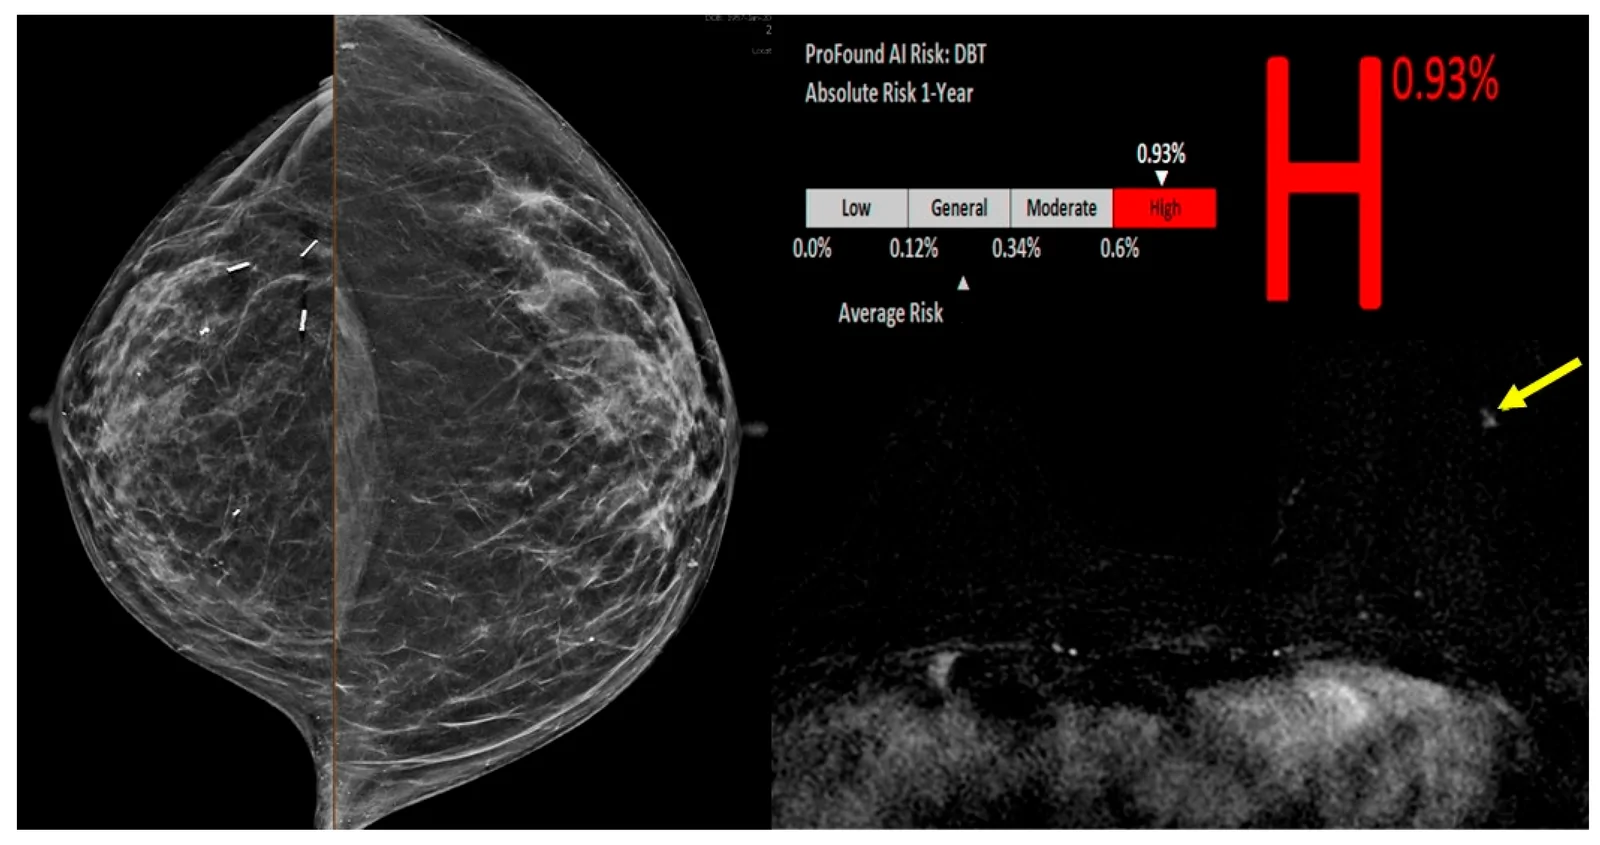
(Subsequent breast cancer [contralateral new primary breast cancer]/Case): 62-year-old BRCA 2 mutation carrier, with a history of right estrogen receptor–positive/progesterone receptor–positive IDC diagnosed 10 years prior, status post lumpectomy and adjuvant radiation. CC images from the index screening mammogram show heterogeneously dense tissue and lumpectomy changes in the right breast assessed as BI-RADS 2. The patient underwent high-risk screening breast MRI six months later, which showed focal non-mass enhancement lesion (yellow arrow) in the outer left breast assessed as BI- RADS 4. MRI-guided biopsy was performed, and pathology revealed DCIS. The AI risk score from the screening mammogram was high. The apparent difference in breast size reflects differences in patient positioning and compression during mammographic acquisition rather than a true anatomical difference.
